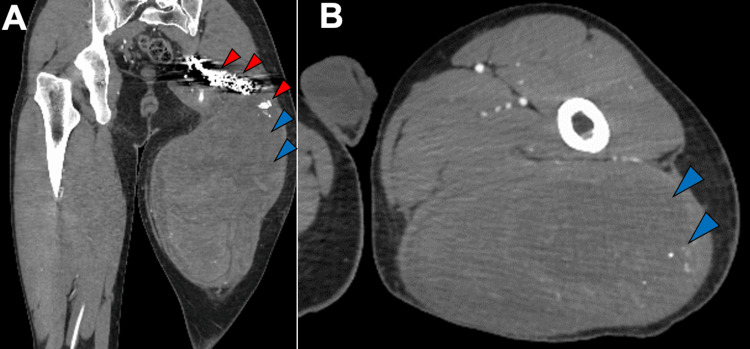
Contrast-enhanced CT obtained three months after the third transcatheter arterial embolization: (A) coronal and (B) axial views. No abnormal vessels or contrast extravasation are detected (panel A and B, blue arrows) within or around the hematoma. Multiple embolization coils (panel A, red arrows) from prior procedures are visible.
